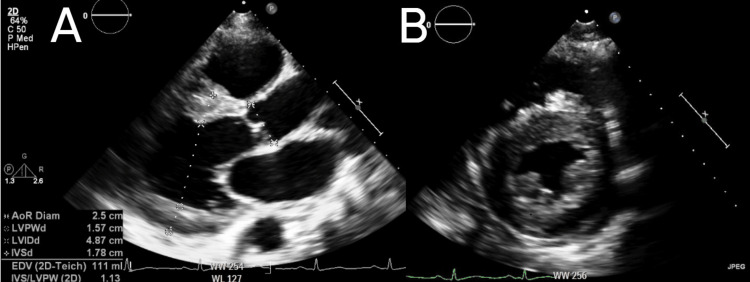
Echocardiogram demonstrating evidence of moderate concentric left ventricular hypertrophy with normal LV cavity size. (A) Parasternal long-axis view on echocardiogram; (B) parasternal short-axis view on echocardiogram. LV: left ventricle.
